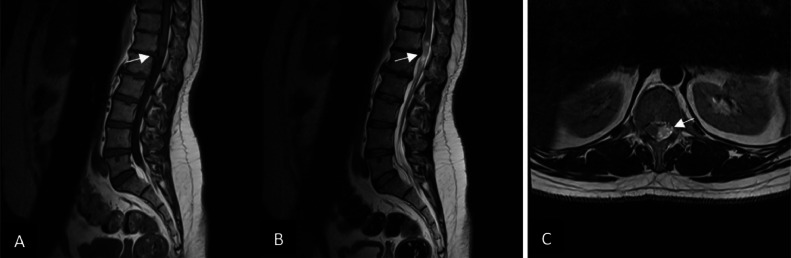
an oval intradural mass lesion is revealed on magnetic resonance imaging of the lumbar spine at the T12-L1 level, with a low signal on T1 W and a high signal on T2 W images marked by short arrows. There is obvious compression of conus medullaris by the mentioned mass.
